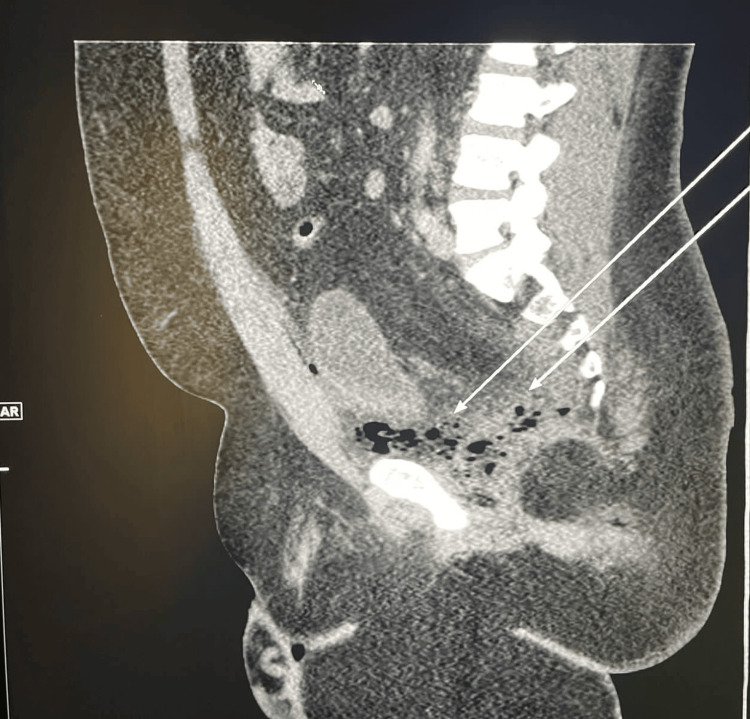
CT scan of the pelvis demonstrating the abscess collection (white arrows).
